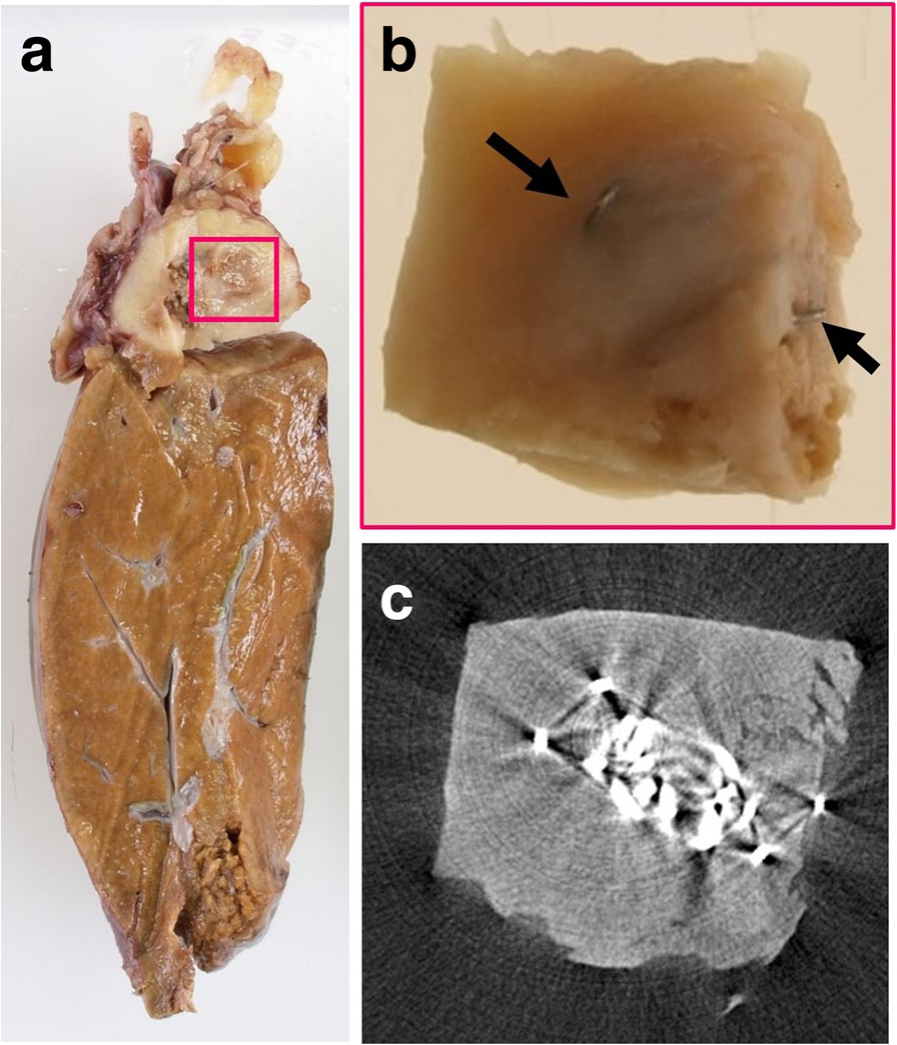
Macroscopic features. a A grey-white solid nodule measuring 45 x 38 mm in the resected liver. b Staple needles in the central portion of the nodule (arrows). c Micro CT
